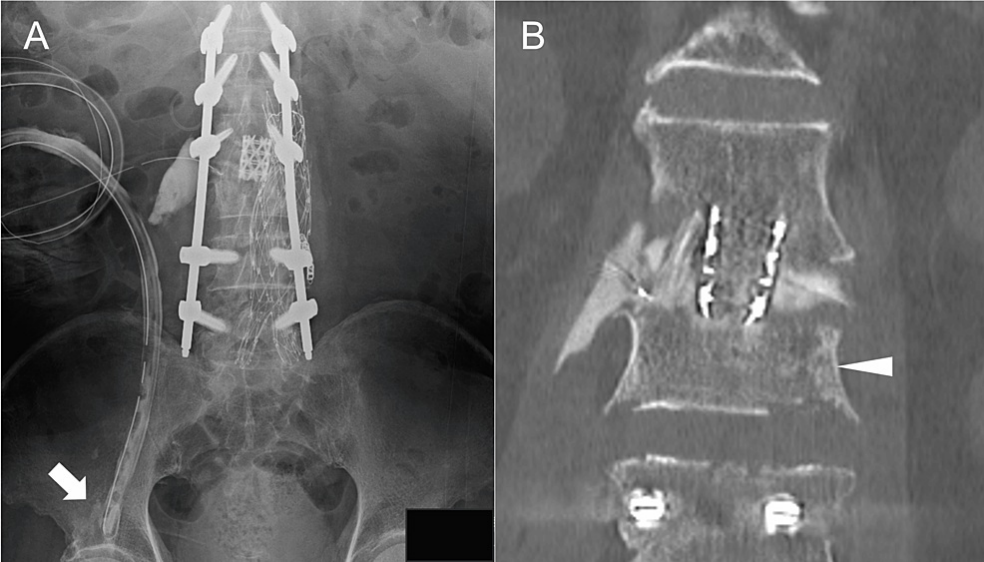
Postoperative contrast test (A) anterior-posterior radiograph, (B) computed tomography A contrast test showing the enhanced small cavity of the iliopsoas abscess (arrow) and the L2-3 intervertebral disc space. Arrowhead: L3 vertebral body
